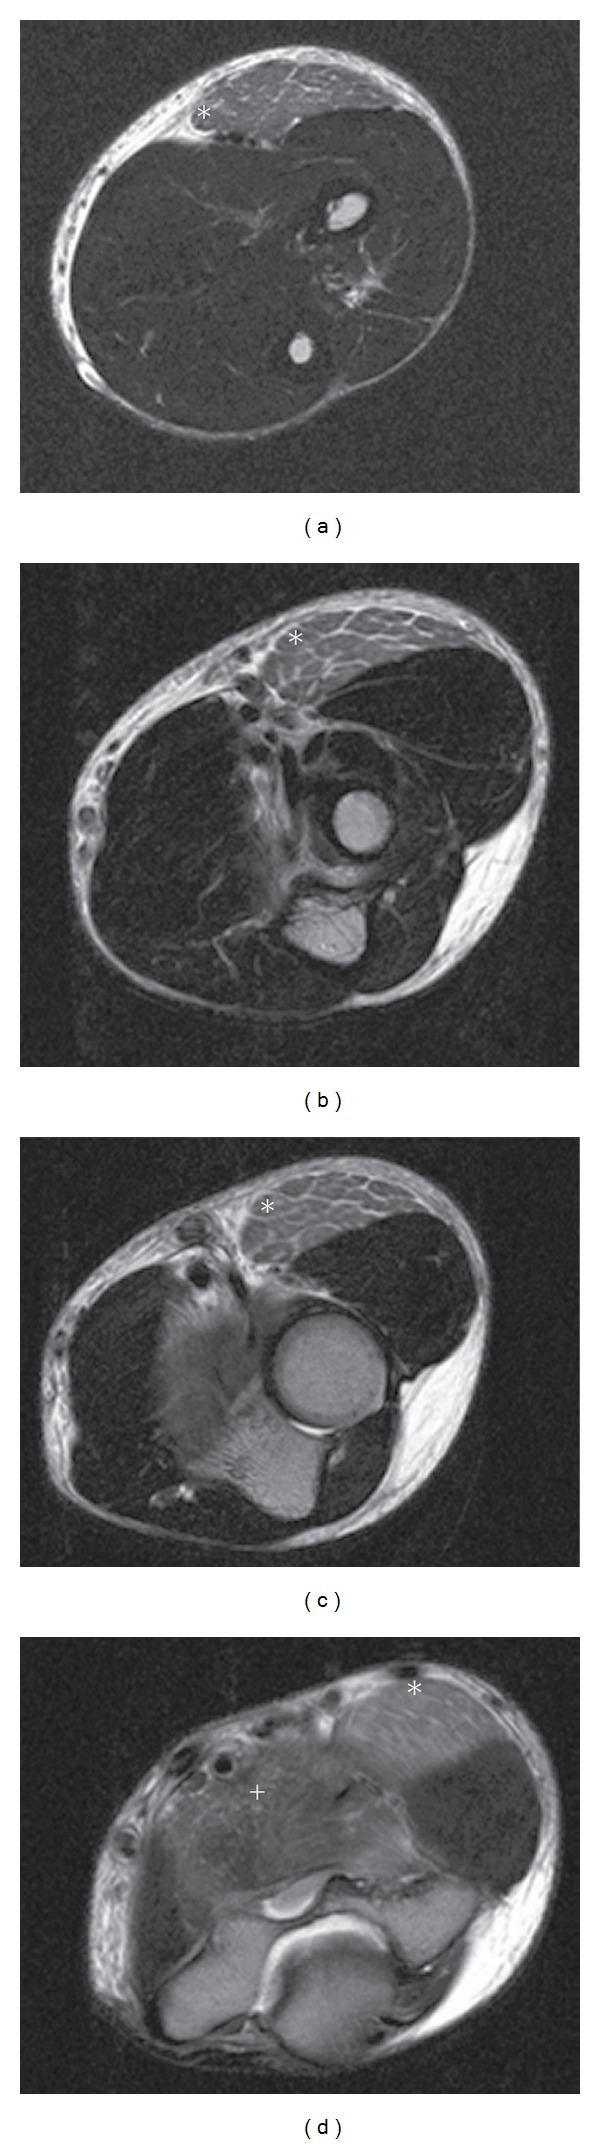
MRI of the right elbow (transversal T2w TSE) shows homogenous signal increase in the brachioradial (marked as *) and the brachial muscle (marked as ^+^).
